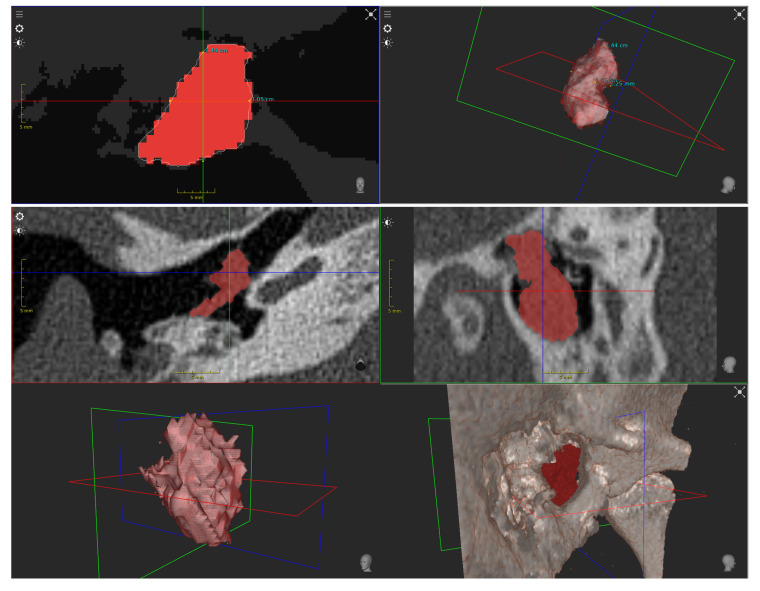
The preoperative CT segmentation from Patient #1 (Top row) and Patient #2 (Middle and Bottom rows) give a first good indicator about the size, shape, and volume of the cholesteatoma.
